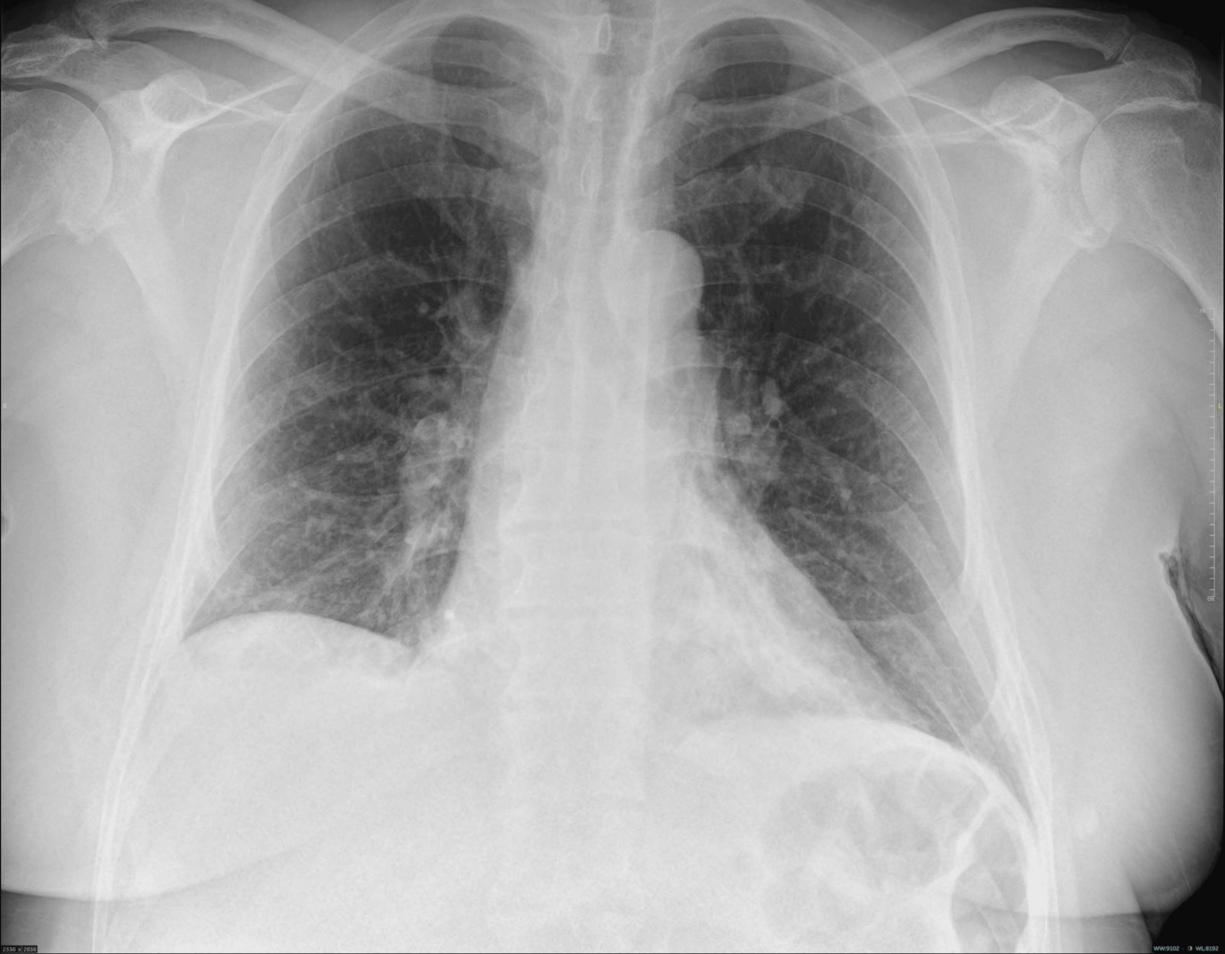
Chest X-ray during current hospital stay.
